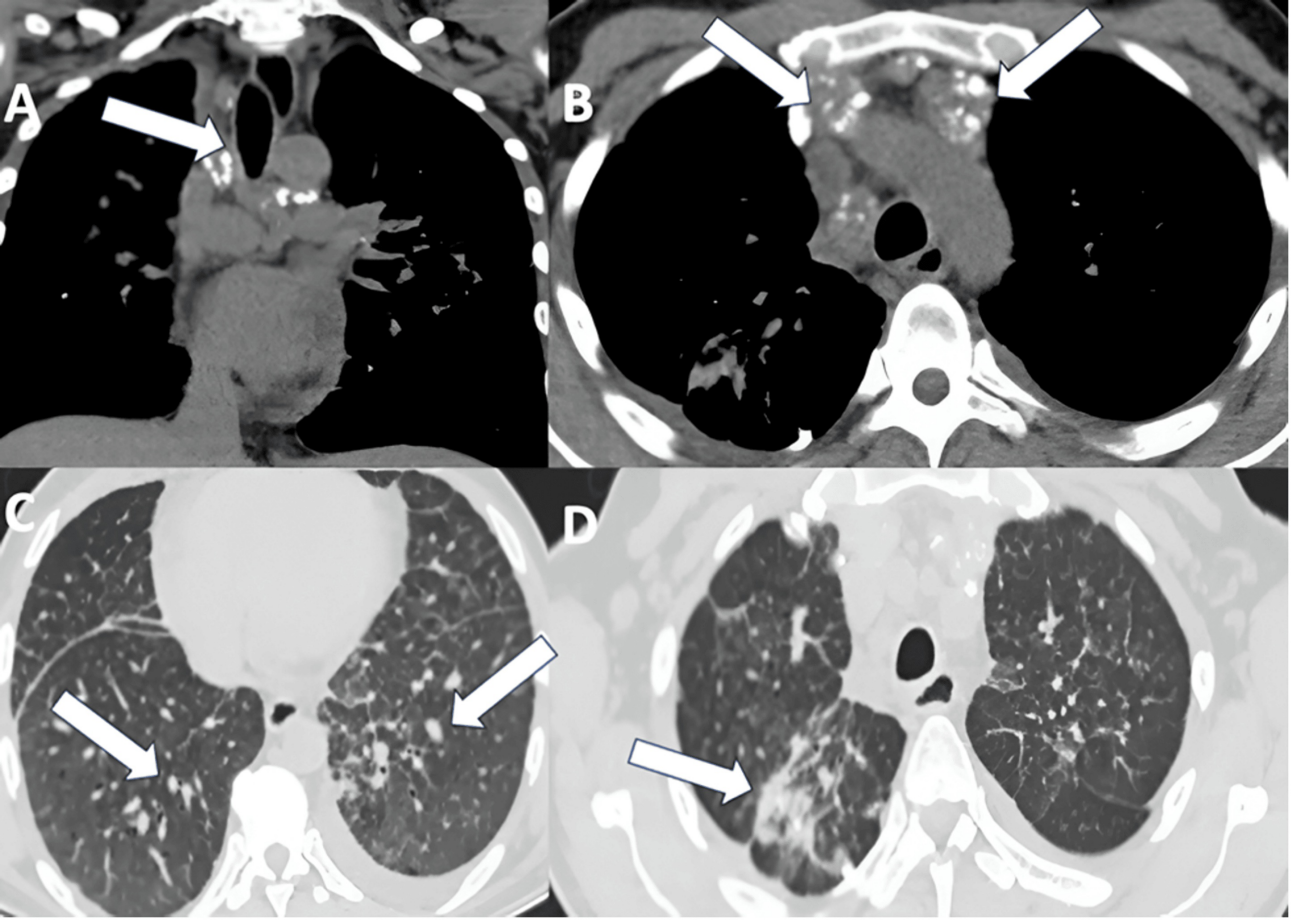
CT chest (A, B) Arrows indicating lymphadenopathy with punctate peripheral calcifications (“eggshell calcifications”). (C) Pulmonary parenchyma with bilateral thickening of interlobular septa and nodular lesions. (D) Pulmonary parenchyma with confluent nodular lesions of irregular morphology in the right upper lobe.
